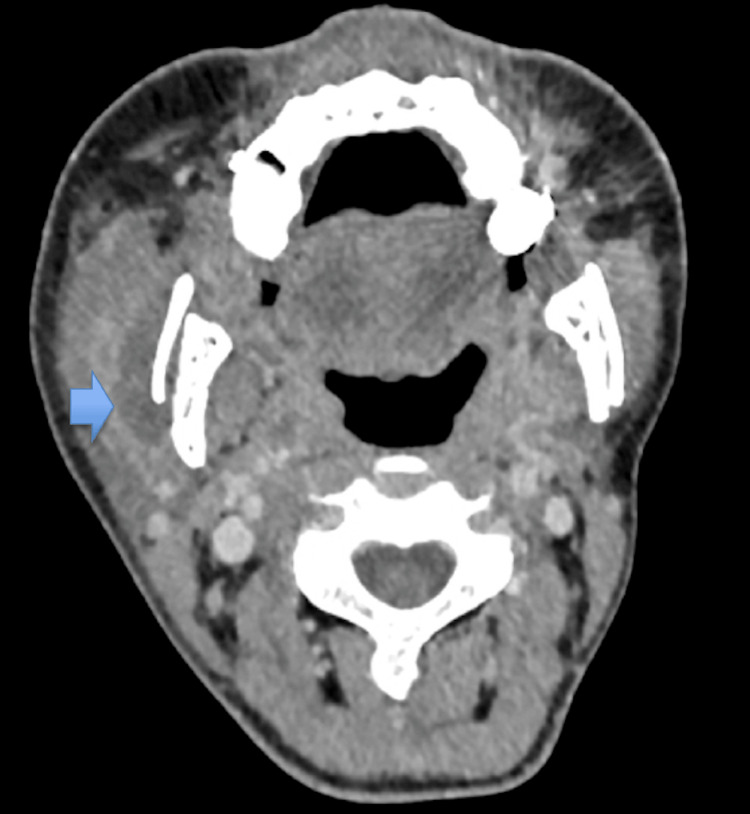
CT image Swelling of the right cheek and a fever of 38.0°C were observed on postoperative day 30. CT images showed a low-density area between the right mandible and the masseter muscle, suggesting fluid accumulation (blue arrows). CT: computed tomography
